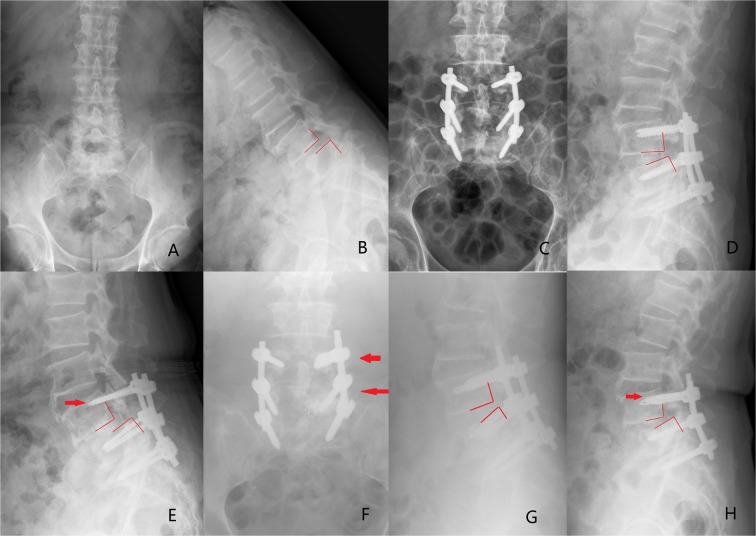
(A,B) A 74-year-old male diagnosed as spondylolysis at the L4 vertebral body with osteoporosis(T = −3.2, degree I). (C,D) Immediate postoperative radiographs showed reconstruction for spondylolisthesis compared with that before surgery. (E) Internal fixation failure occurred 1 month after surgery. Lateral radiograph showed L4 vertebral body was displaced again and the red arrow showed screw loosening and pullout. Patient redeveloped the lumbocrural pain. (F,G) After treatment with screw tunnel augmentation using PMMA (left side of L4 and L5, as the red arrows showed), L4 vertebral body was reset again. (H) One month after the revision surgery, however, screw failure occurred again (the red arrows showed the PMMA augmentation screw loosening).
